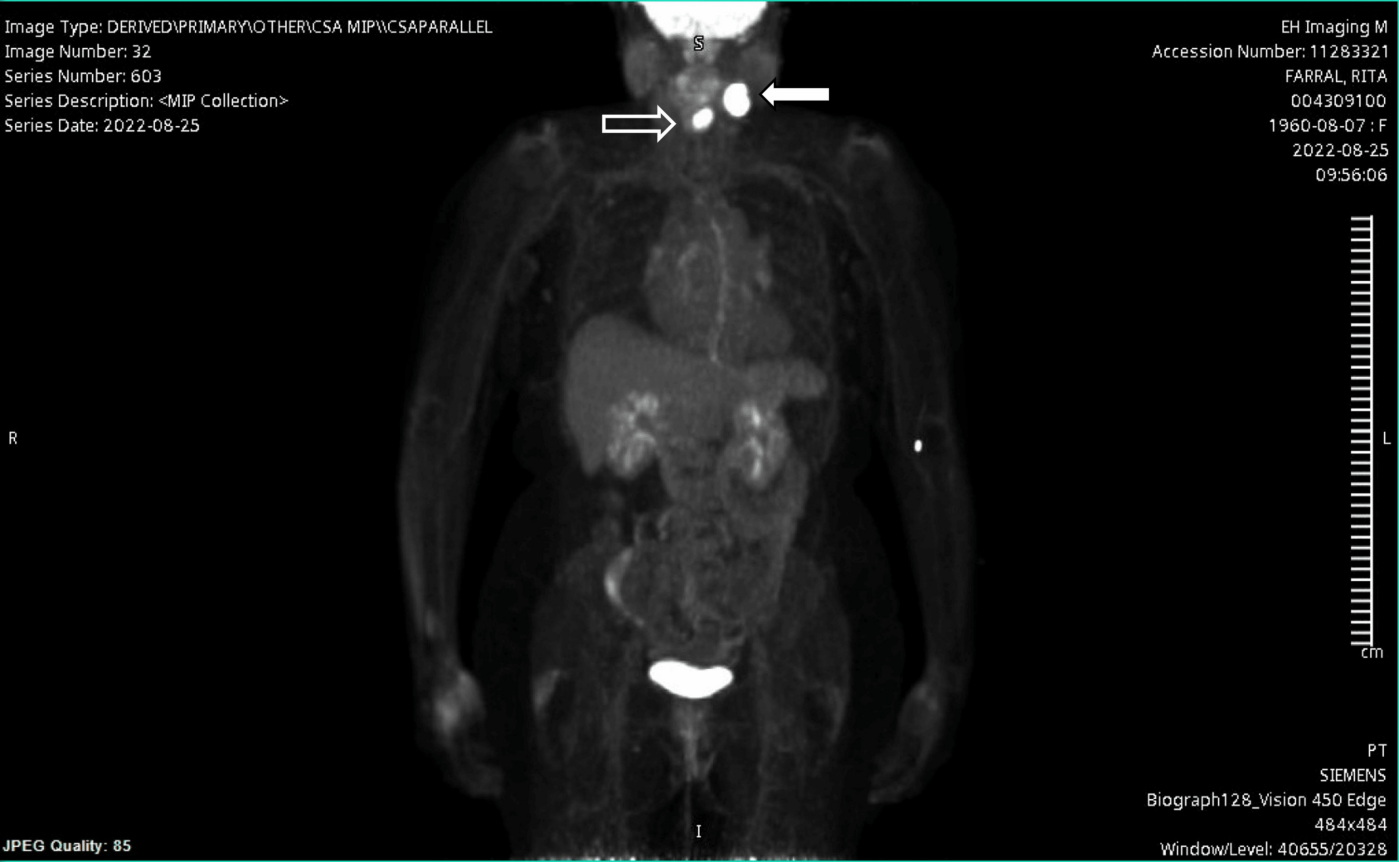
PET-CT of the laryngeal carcinoma and the neck mass This is the PET-CT of the laryngeal mass (open arrow) and the cervical neck mass (white arrow). These results confirm the laryngeal carcinoma location and demonstrate no other malignant spread other than the cervical lesion identified on PET-CT imaging. Identifying local spread helps determine treatment options for the treating surgery and oncology teams.
